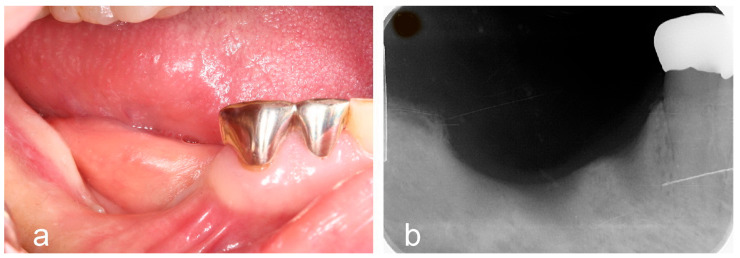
Preoperative intraoral photograph and periapical radiograph. (a) Clinical view showing a severe vertical soft and hard tissue defect following implant removal due to peri-implantitis. (b) Radiographic image confirming the presence of a substantial vertical bone defect in the edentulous ridge.
